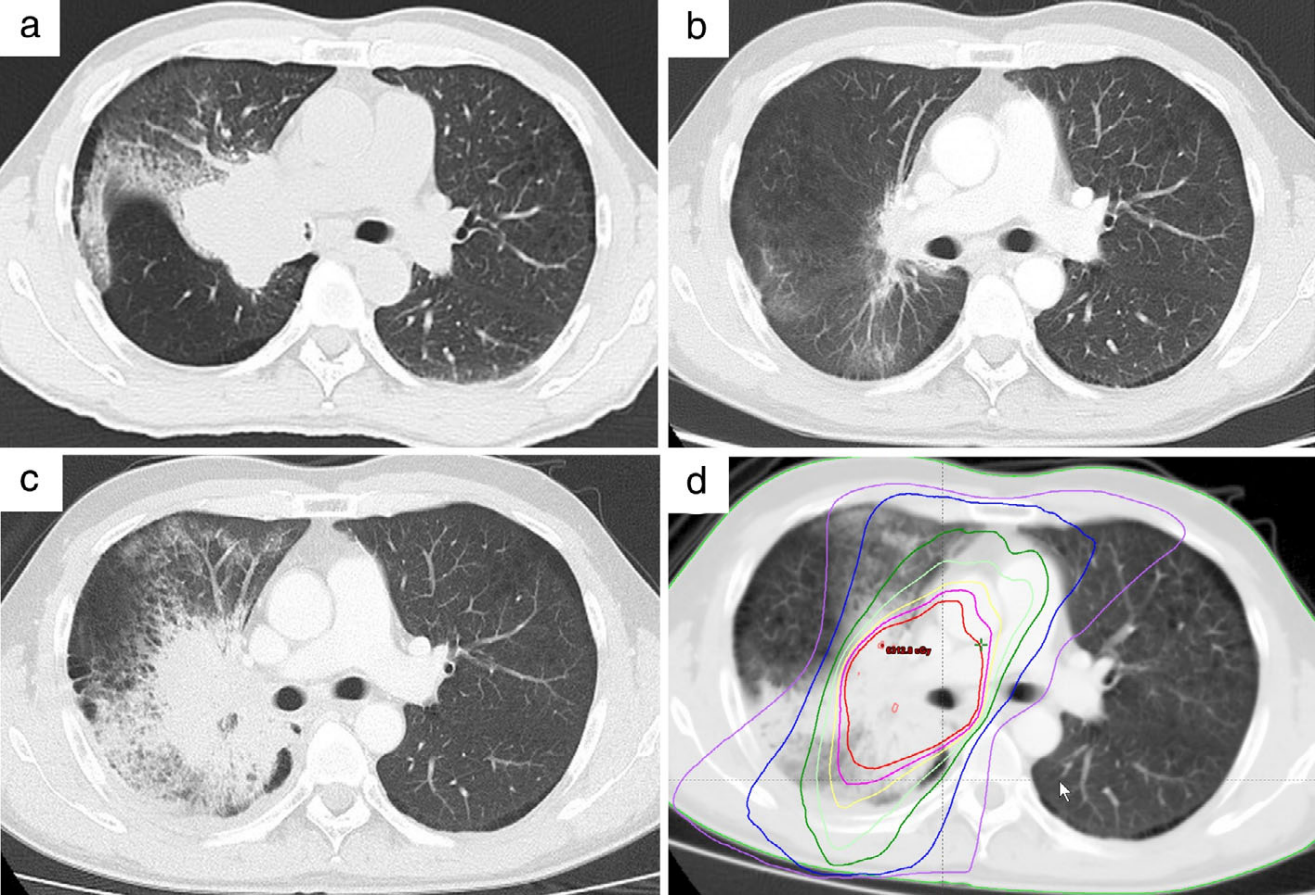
Computed tomography (CT) images of pulmonary opacity during the clinical course of the patient. (a) CT scan at diagnosis revealed a mass lesion in the right lower lobe. (b) There was no evidence of pneumonitis on the CT scan after the completion of durvalumab maintenance treatment following concurrent chemoradiotherapy. (c) Twenty‐one days after the second COVID‐19 vaccination, suspected radiation recall pneumonitis was detected. (d) Comparison of the planned isodose curve in the treatment plan and pneumonitis
